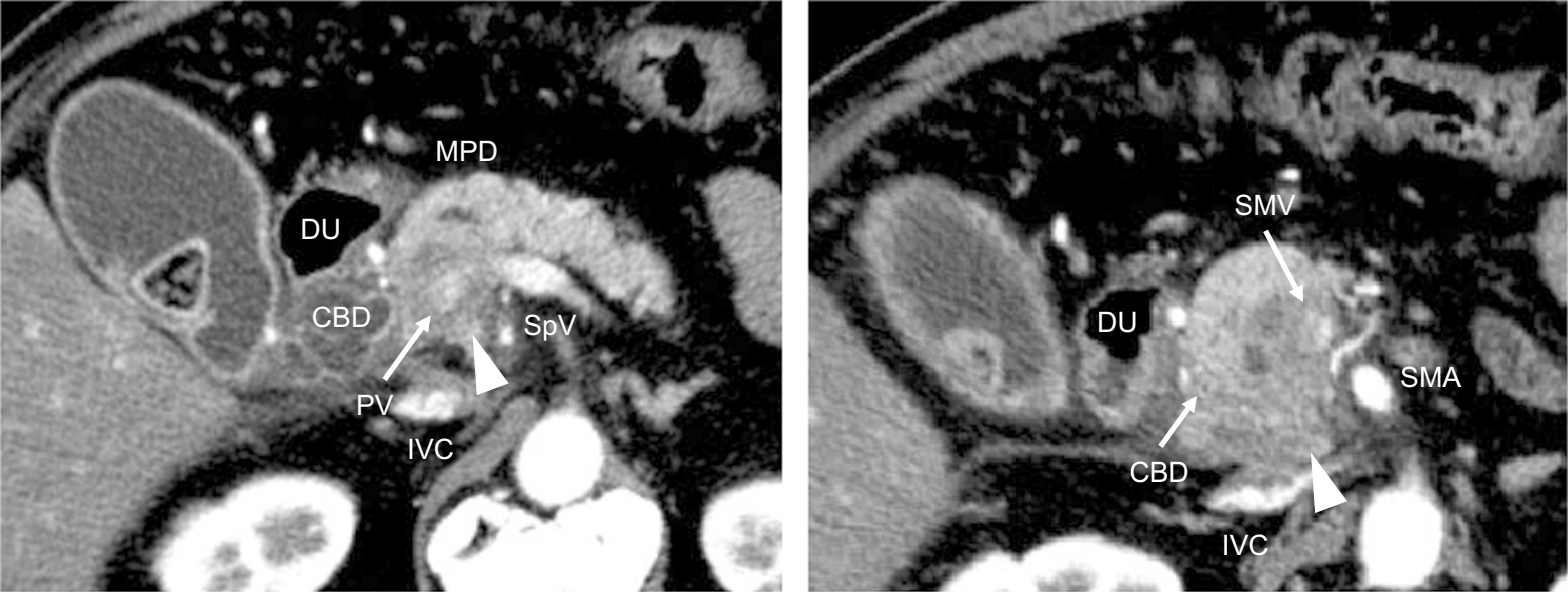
Preoperative enhanced computed tomography (CT). Abdominal axial CT shows a 48-mm hypo-vascular mass in the pancreatic head (arrowhead) involving the superior mesenteric vein and portal vein all around (arrow)
